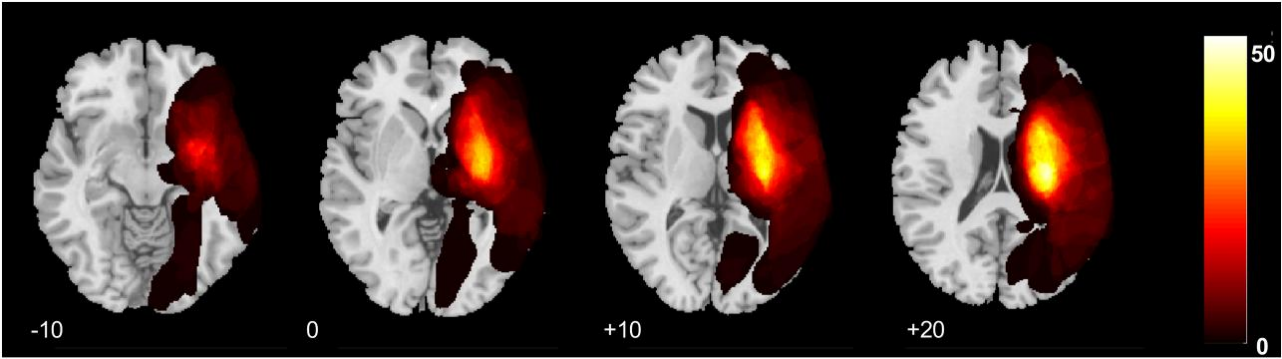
Lesion overlap map of the study cohort. Overlapping lesion map of the study cohort on an MNI template. Numbers correspond to the coordinate of the axial slice in the MNI space. Colour scale bar: number of patients with lesions in a particular region. The lesion outline of each patient was based on T_1_-weighted MRI acquired in the sub-acute stage. The right side of the images represents the right side of the brain (here and in the following MRI Figures) (*N* = 114).
